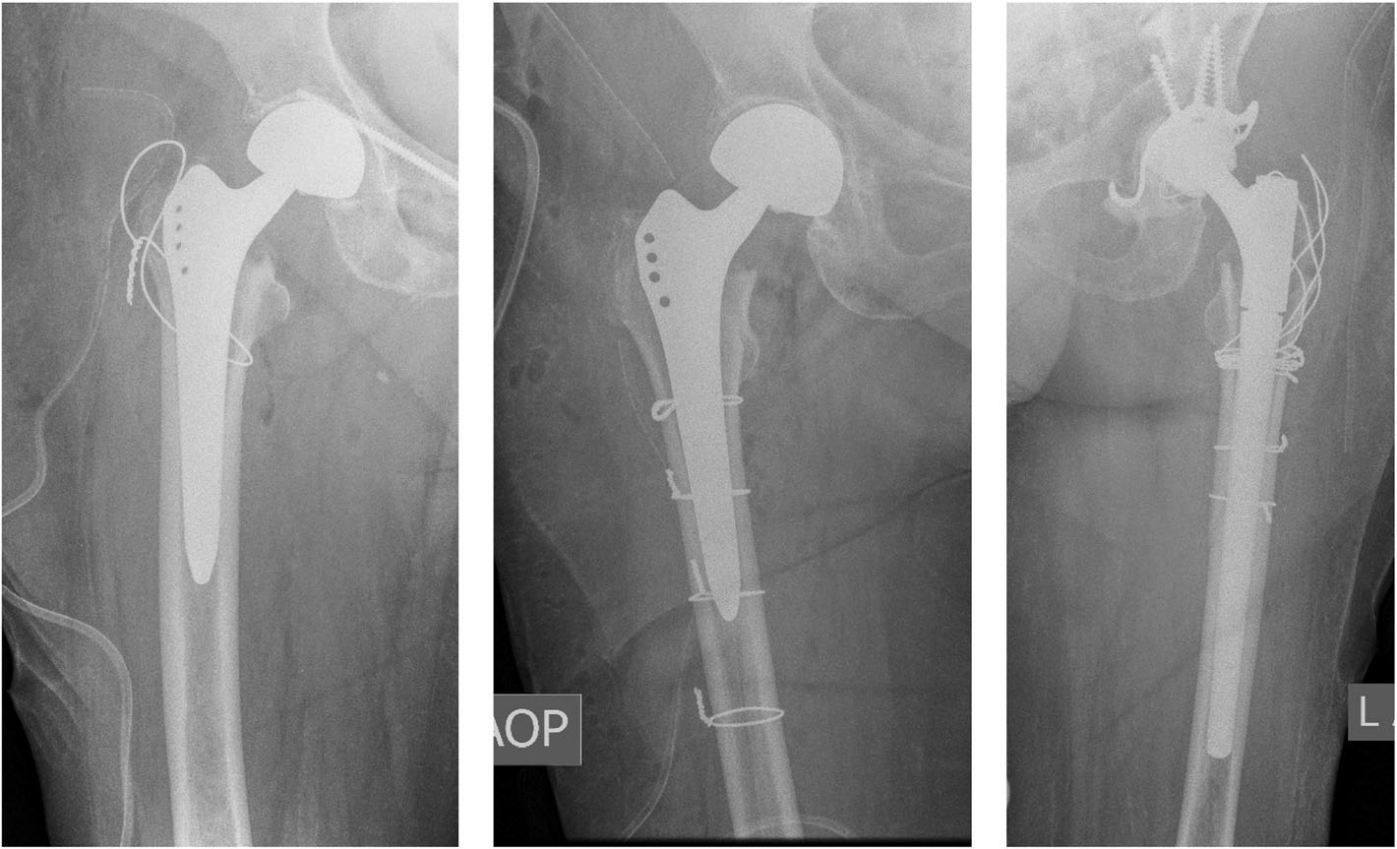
Salvage treatments for IPF. The panel labels depict the side shown on the radiographs. a Figure of 8 cerclage wire. b Single loop cerclage wires around shaft area. c Revision arthroplasty after postoperative implant failure following IPF
